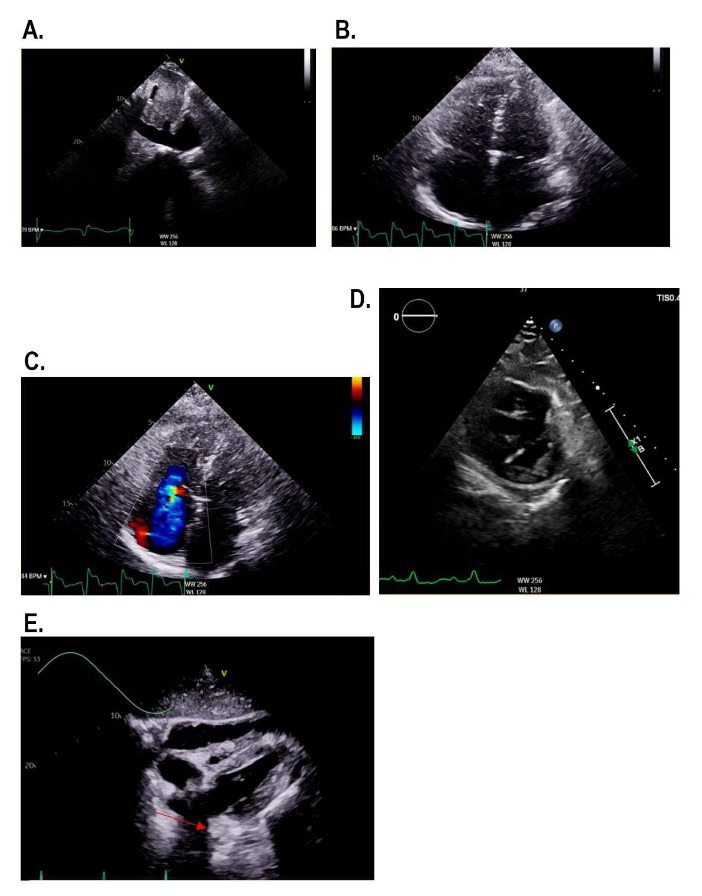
Abnormal point of care ultrasound (POCUS) scans of patients with PH A. Inferior Vena Cava (IVC) Dilatation. B. Right Ventricle (RV) Dilatation. C. Severe Tricuspid Regurgitation. D. Dilated RV in the parasternal short axis. The D-shaped septum is indicative of RV pressure overload. E. Pericardial effusion.
